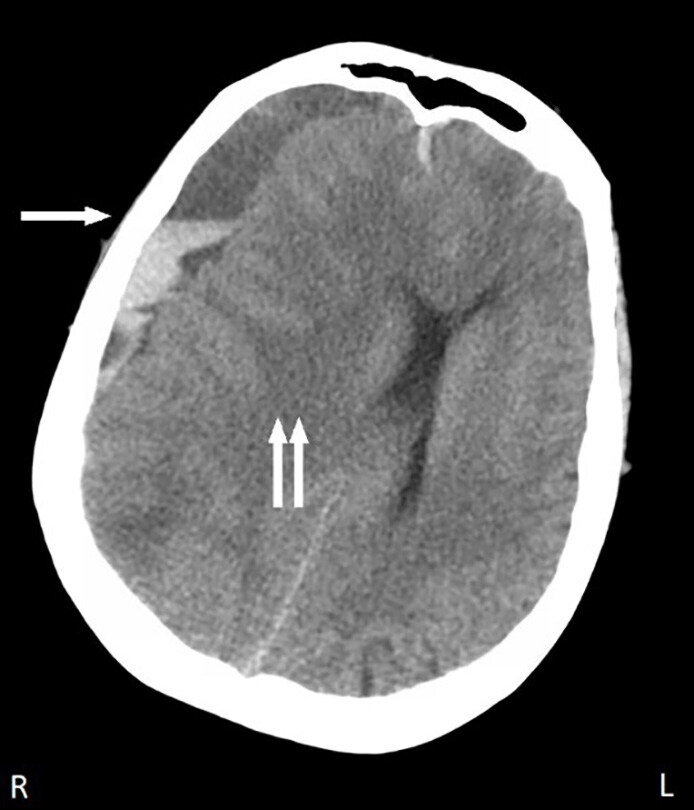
Axial view of noncontrast computed tomography of the brain depicting a right-sided acute-on-chronic subdural hematoma (arrow) with right-to-left midline shift and ventricular obliteration (double arrow) in a 64-year-old female with history of non-small-cell lung cancer following initiation of therapeutic heparin anticoagulation for treatment of deep venous thrombosis.
